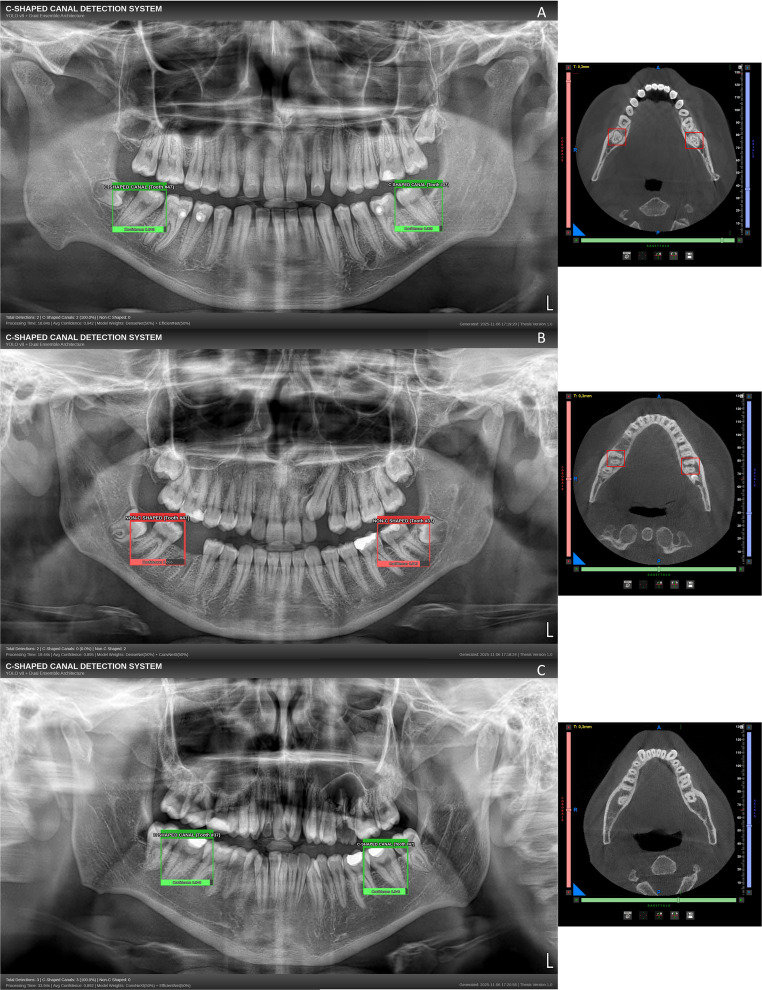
Dual ensemble classifier outputs on panoramic radiographs (left) with the corresponding CBCT horizontal slices used as the reference standard (right). (A) DenseNet-169 + EfficientNet-B6, (B) DenseNet-169 + ConvNeXt-Base, (C) ConvNeXt-Base + EfficientNet-B6. On the panoramic images, green boxes indicate the presence and red boxes the absence of a C-shaped canal; all boxes reflect YOLOv8-based localization of the target teeth. On the CBCT panels, the red boxes delineate the mandibular second molars (#37 and #47) used for ground-truth adjudication
